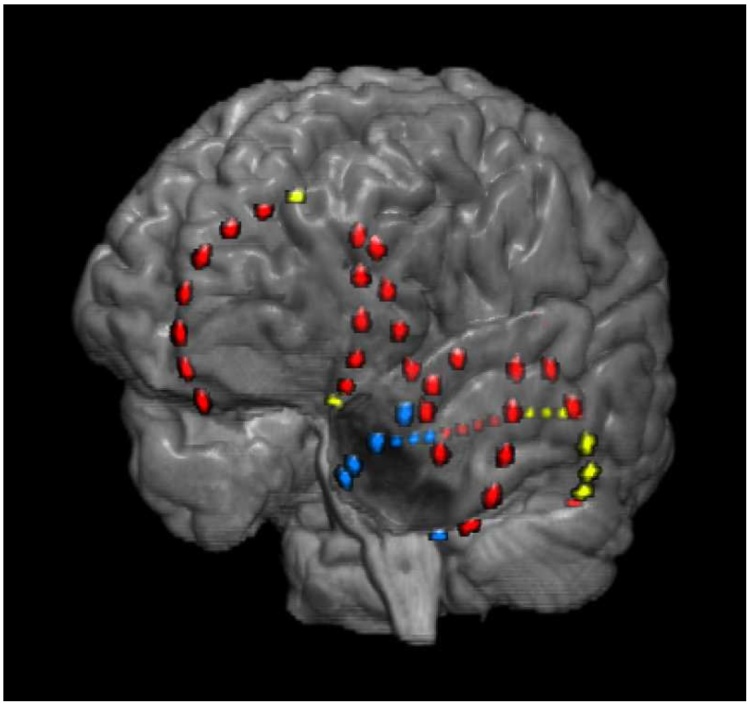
Representation of the actual resection of class IV patient 16 and a hypothetical resection assessed by both methods as highly beneficial. A pre-surgical MR recording was coregistered with a post-implantation CT recording do determine the position of the iEEG-electrodes (colored dots). The channels removed during the actual resection are located around the temporal pole (blue dots) whereas the hypothetical resection is mainly located in the posterior temporal lobe (yellow dots). (For interpretation of the references to colour in this figure legend, the reader is referred to the web version of this article.)
